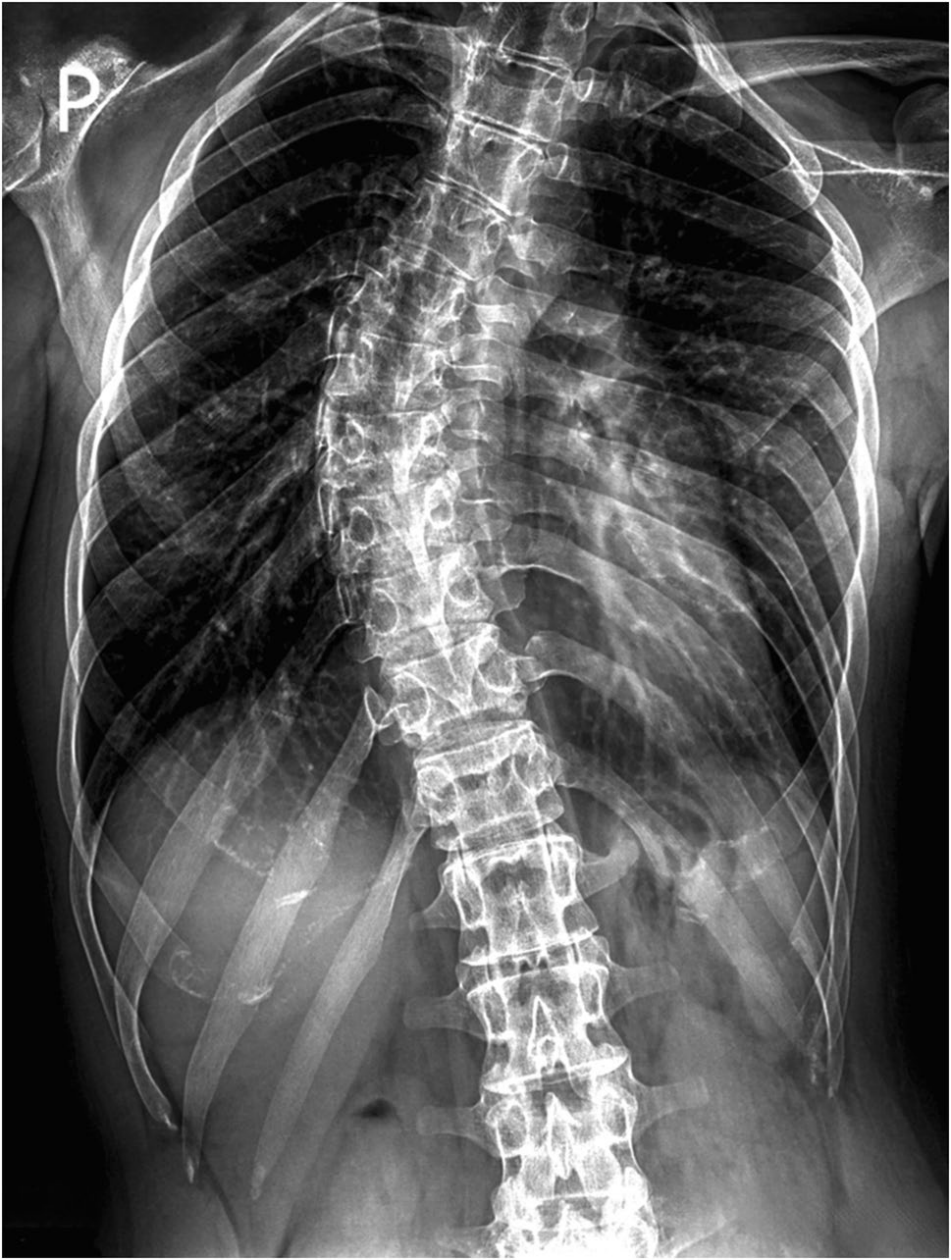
The spine x-ray shows thoracic scoliosis in patient 2 with *TBCE* c.100 + 1G > A homozygous variant
